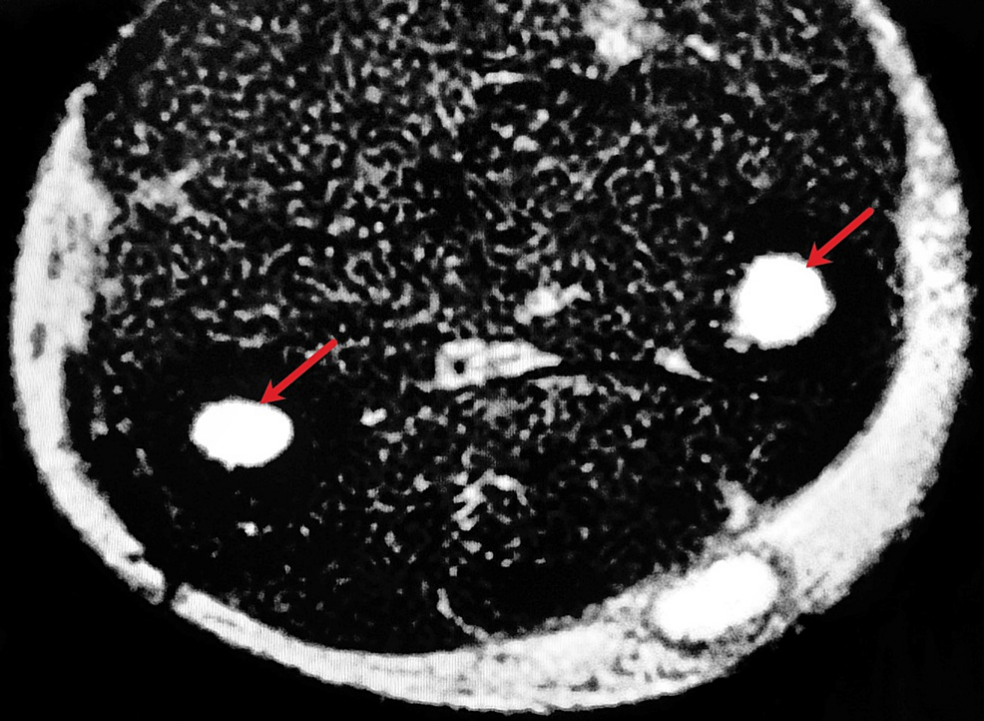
Magnetic resonance imaging A subcutaneous hyperintense circumscribed lesion with two structures (red arrows) within the lesion
